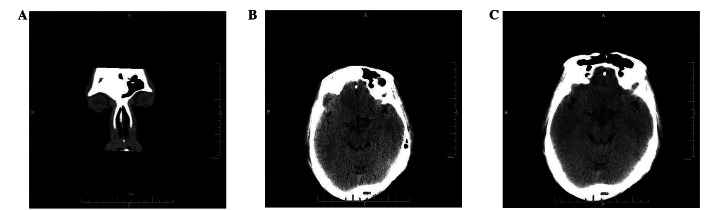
(A) A computed tomography (CT) scan demonstrated a giant bone tumor filling in the right frontal sinus and pushing into the left frontal sinus in coronal CT images. (B) A CT scan demonstrated a giant bone tumor filling the right frontal sinus and pushing into the left frontal sinus in axial CT images. (C) The CT scan revealed total resection of the osteoma 3 months after the surgery in axial CT images.
